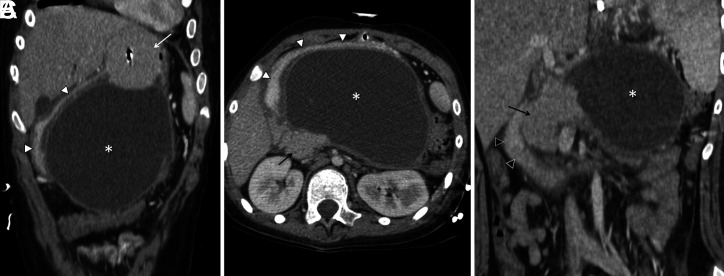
CT images of the patient diagnosed with gastric outlet obstruction and pancreatitis. On contrast-enhanced axial (B) and coronal reformatted (A, C) abdomen CT images, a lobulated, contoured, thin-walled cystic lesion (*) measuring 135 × 88 × 130 mm in the widest part and involving the body and tail of the pancreas is seen. Gastric lumen (white arrowheads) and PEG balloon (white arrow) can be distinguished superior to the cyst, and the pancreatic head and neck (black arrow) can be seen in the lateral aspect of the cyst. On the lateral side of the pancreatic head, we can also discern the natural course of the second and third portions of the duodenum (black arrowheads). CT, computed tomography; PEG, percutaneous endoscopic gastrostomy.
